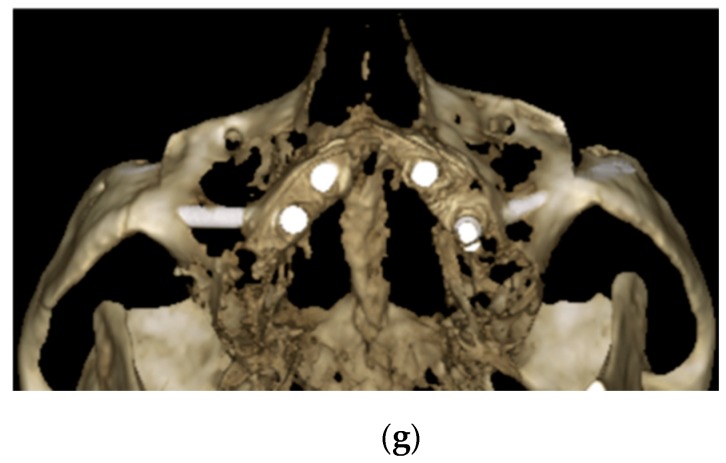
(a) Atrophic alveolar bone crest seen in a preoperative three-dimensional reconstruction CBCT image. (b) Atrophy of edentulous alveolar crest seen in an intraoral clinical image. (c) Placement of two posterior zygomatic implants (Branemark System Zygoma^®^, Nobel Biocare, Sweden). The coronal part of the zygomatic implant remains in a palatal position, and buccal fenestration is presented. (d) Buccal-centered image showing the buccal and palatal bone graft (KeraOs^®^, Keramat, Spain)). (e) Buccal-centered image showing overlap of the resorbable collagen membrane (Creos Xenoprotect^®^, Nobel Biocare) upon the bone graft. (f) Soft tissue healing at one month after implant placement. (g) Postoperative three-dimensional reconstruction CBCT image showing palatal and buccal bone regeneration around the implants at 6 months after surgery.
